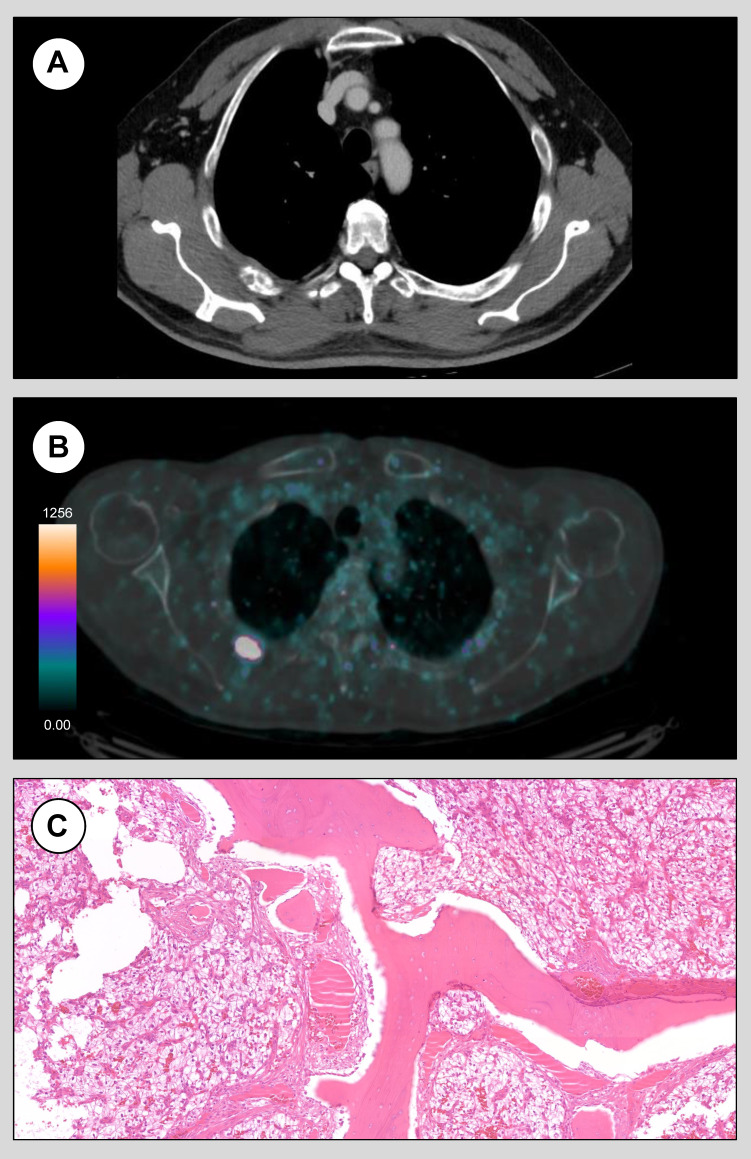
Axial standard chest CT images (A) and CAIX-PET images (B) of case 2. CAIX-PET prompted the reclassification from cM0 to cM1 by detecting pathological radiopharmaceutical uptake in the fourth rib. Following surgical metastasectomy, final pathology confirmed the presence of osseous trabeculae interspersed with richly vascularized neoplastic tissue composed of cells with abundant clear cytoplasm, consistent with metastatic ccRCC.
